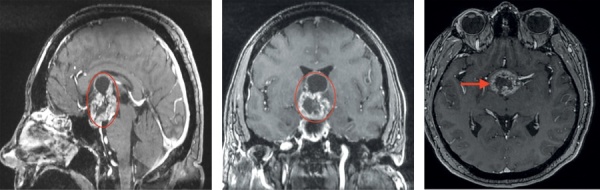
Рисунок 1. МРТ пациентки З. до операции. Визуализируется солидно-кистозная супраселлярная краниофарингиома с интравентрикулярным распространением, вовлечением стебля гипофиза (обозначена и указана стрелкой).Figure 1. MRI of patient Z. before surgery. A solid cystic suprasellar craniopharyngioma with intraventricular extension involving the pituitary stalk is visualized (marked and indicated by an arrow).
